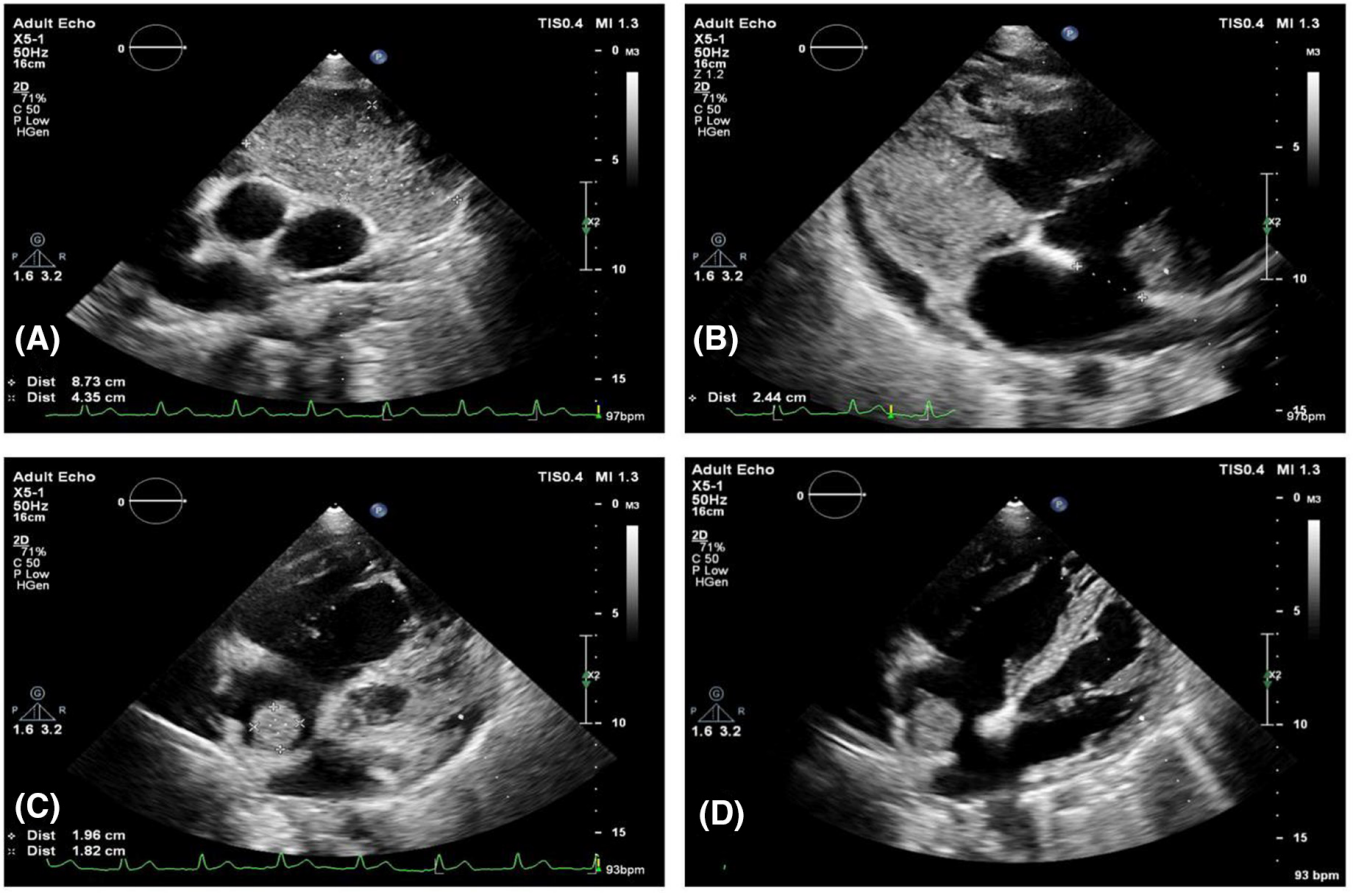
Echocardiography. Evidence of large anterior to ascending aorta (A). Large ASD secundum type, 2.4 cm (B). Large nonmobile mass in RA, attached to RA wall (C, D).
